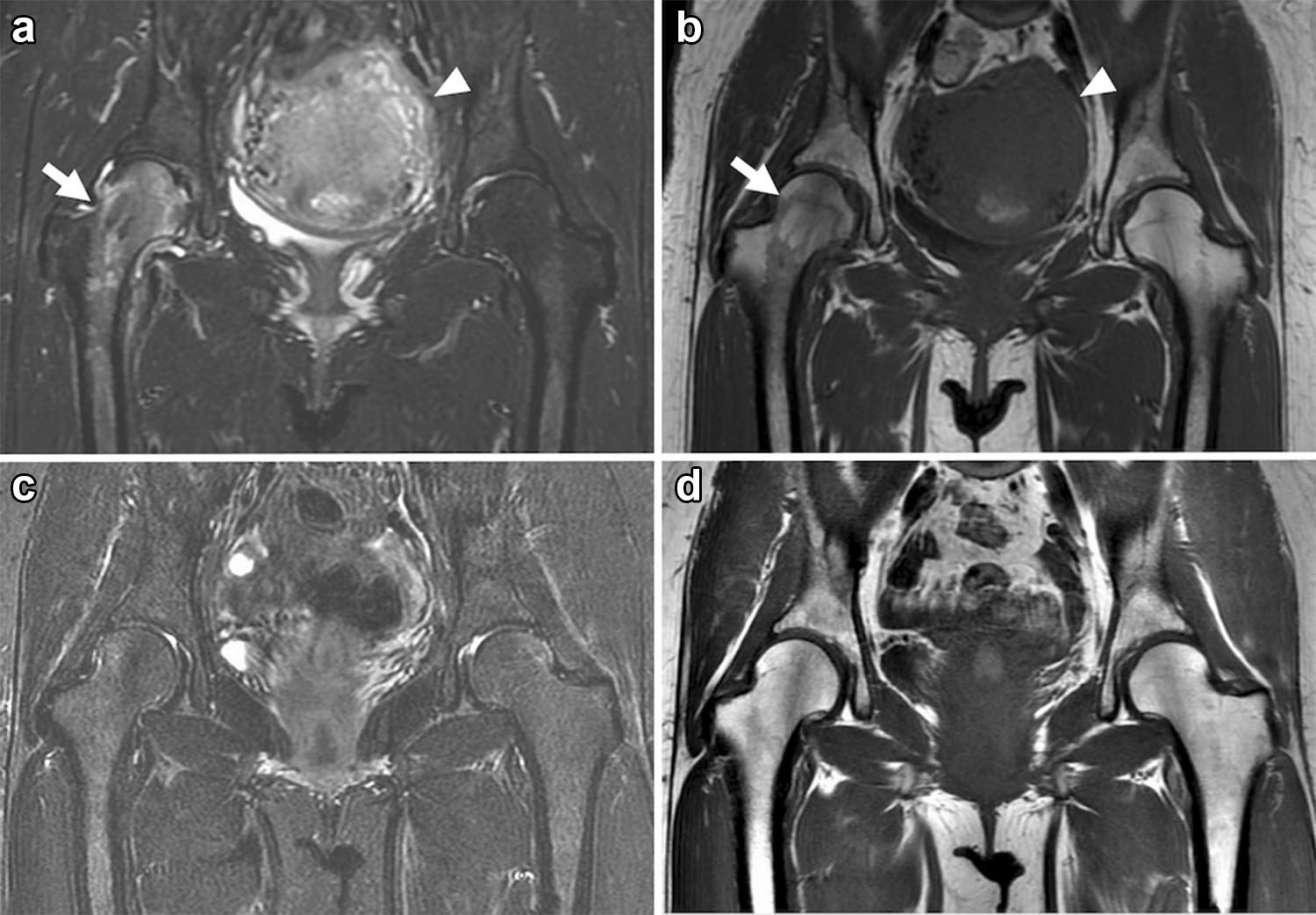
Coronal FS-PDWI and T1WI of a 40-year-old female patient in the postpartum period with transient BMES in the right hip. MRI was performed on the 12th day post-cesarean section for the complaint of severe right hip pain. a, b Initial MRI shows a diffuse bone marrow edema-like lesion extending from the right femoral head to the trochanteric region (arrow). No other obvious abnormal findings are noted, and the postpartum uterus is still enlarged (arrowhead). c, d The patient was treated conservatively, and her symptoms improved. A follow-up MRI taken 4 months later shows the disappearance of the bone marrow edema-like lesion in the right hip
